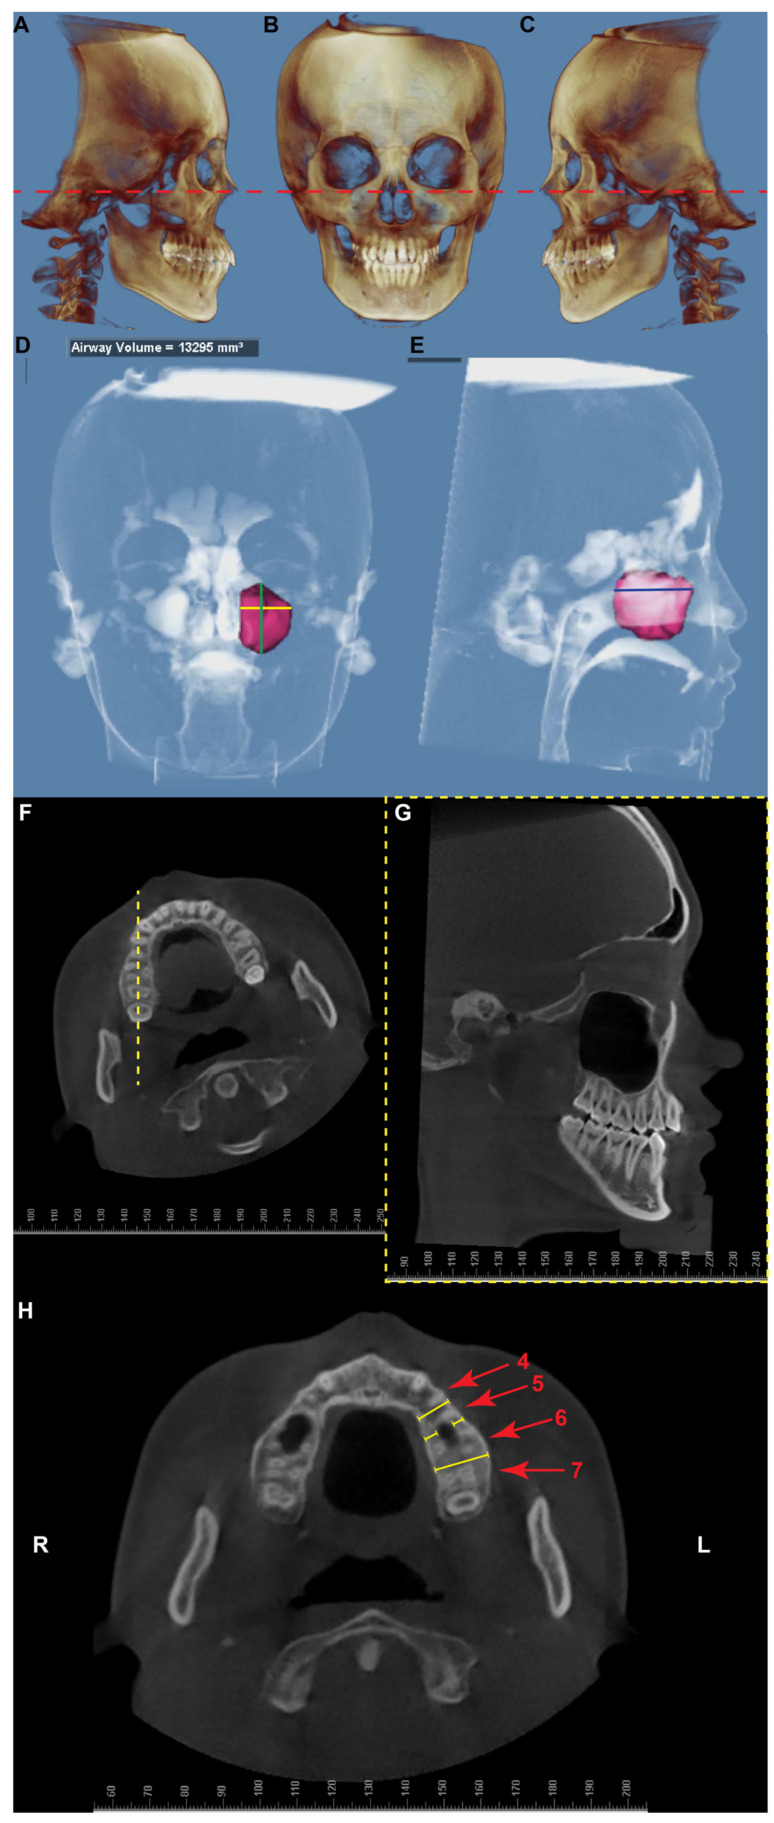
The demography of CBCT measurement protocol used in the current study. (A–C) The CBCT image was oriented to Frankfort horizontal plane (red dash line) using right and left porions (A, front view) and the right orbitale (B, right view), then verified on the left view (C). (D) Dolphin Software 3D airway/sinus rendering module was utilized to measure the volume of the maxillary sinuses. The sinus volume was directly reported by the software. The image showed the left maxillary sinus of this patient. The height (solid green line) and width (solid yellow line) of the maxillary sinus were measured on the front view. (E) The depth of the maxillary sinus (solid blue line) was measured on the side view. (F) The CBCT image was oriented to have the sagittal cut (yellow dash line) midway between the buccal and palatal cortices for the alveolar bone height measurement between every two posterior teeth. (G) The sagittal view generated from the orientation shown in panel F for the alveolar bone height measurement between every two posterior teeth. (H) The demography of alveolar bone thickness measurement buccally and palatally to the maxillary sinus on the left side of the patient. An axial slice of a CBCT image at the level apical to the maxillary posterior alveolar bony crest. The red numbers and arrows indicate the roots of the posterior teeth—4, first premolar; 5, second premolar; 6, first molar; 7, second molar. The alveolar bone thickness measurements on this slice were marked as yellow lines. Between the right first and second premolars, and between the first and second molars, no maxillary sinus penetration was noticed. Thus, the measurements were made from the buccal cortex to the palatal cortex in this location to represent the alveolar bone thickness—R, right side; L, left side.
